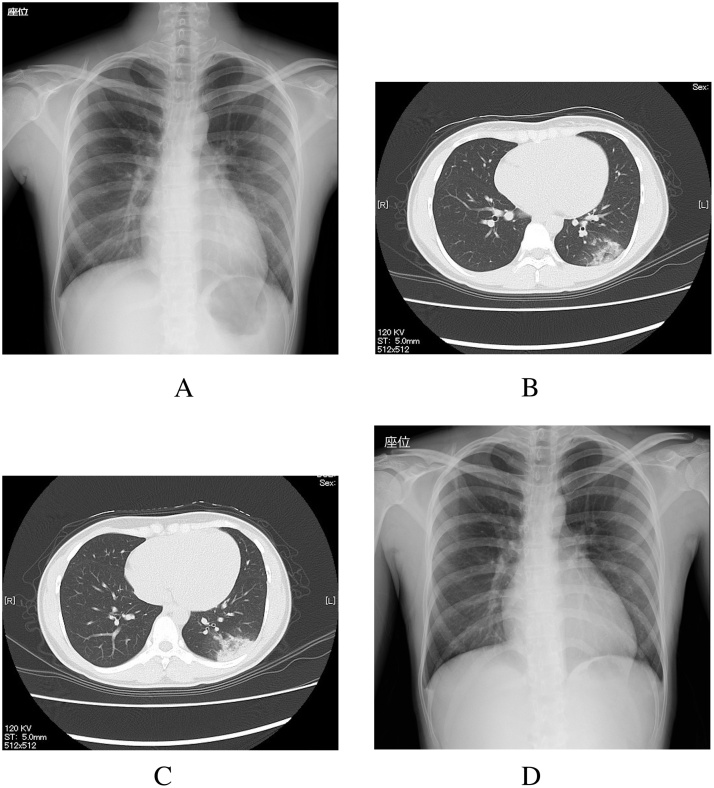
Chest imaging showing signs of COVID-19. A: Chest X-ray showing an abnormal shadow in the left lower zone on admission. B, C: Chest computed tomography showing a patchy infiltrative shadow in the left lower lobe. D: Chest X-ray showing improvement of the abnormal shadow on the day 8.
